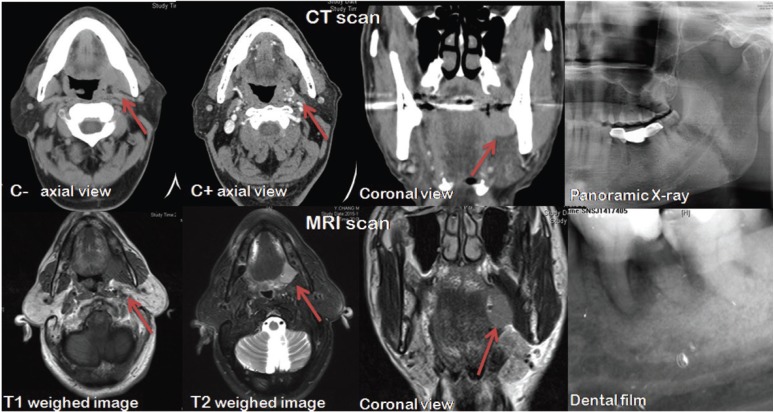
Representative image examination (CT images, MRI images, panoramic X-ray and dental film) of one case of peripheral ameloblastoma.
